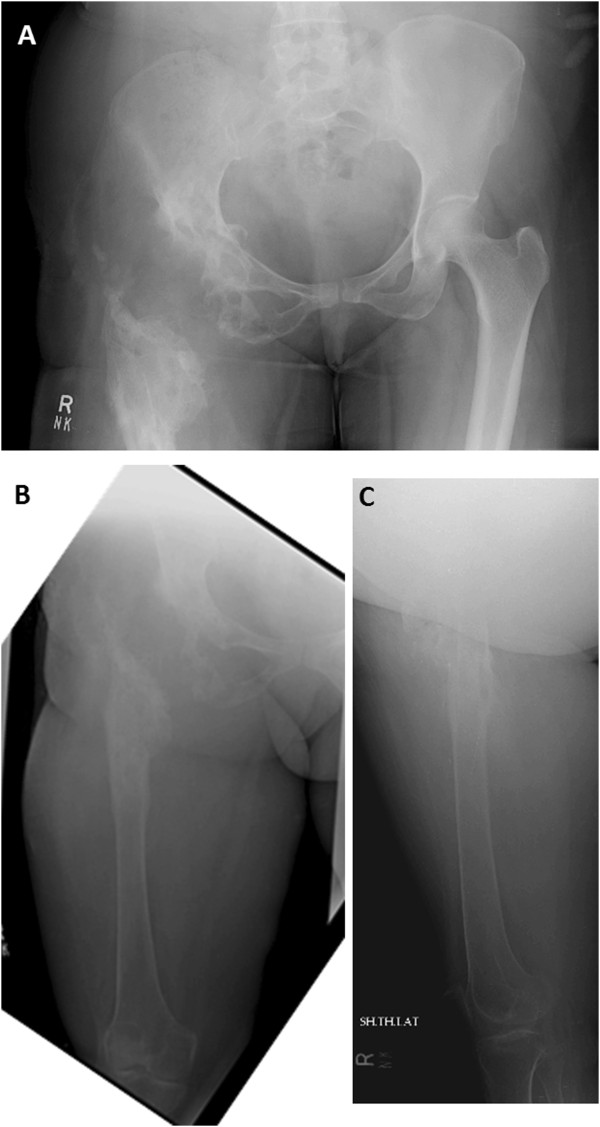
X-ray pelvis (Figure 6A) and femur (Figure 6B and 6C) on presentation at our institute in 2009. There is bone loss in proximal femur with extensive involvement of proximal femur shaft and presence of radiolucencies in acetabulum as well.
